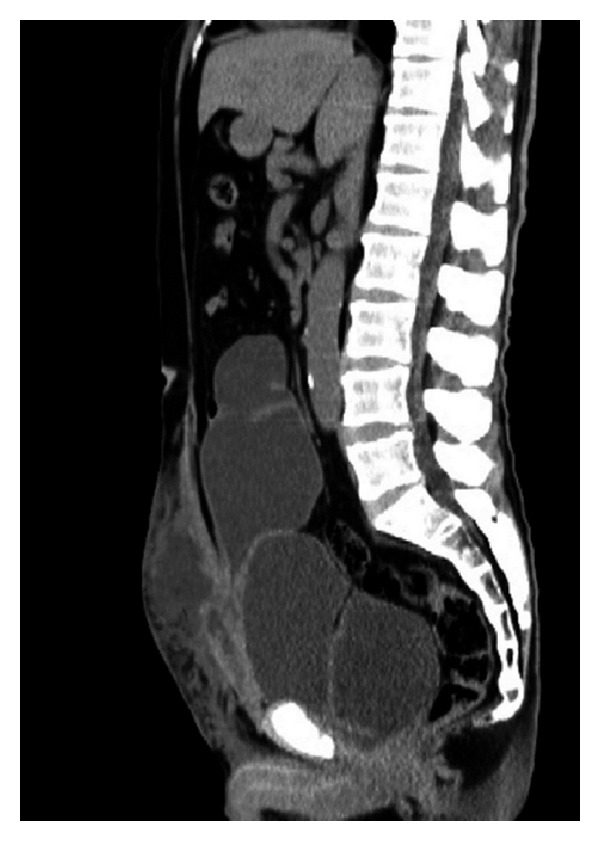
Sagittal unenhanced computed tomography images of the abdomen showing a distended neobladder and subcutaneous fluid accumulation in the lower abdomen. There is no evidence of ascites, intraperitoneal free gas, or incisional herniation.
